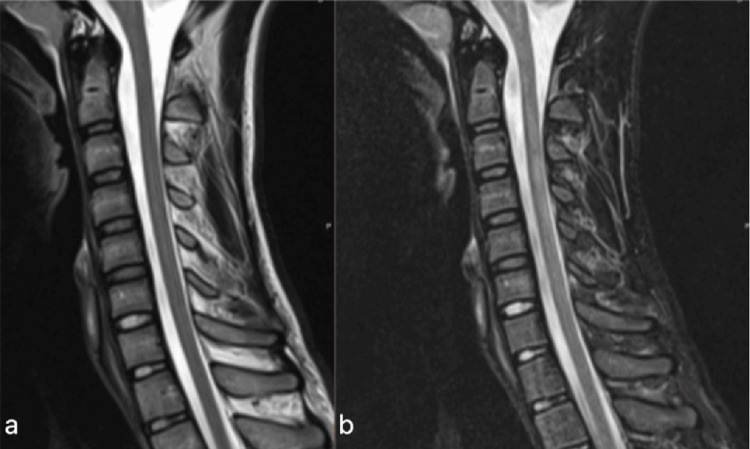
Cervical MRI scan showing no appreciable changes. a: T2 sagital. b: short tau inversion recovery sagittal
